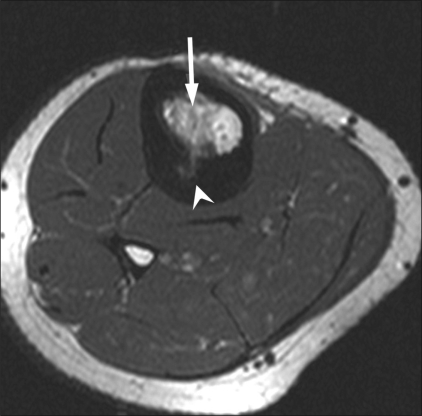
Osteoid osteoma of the tibia. Postprocedure T1W axial MRI shows the zone of ablation (arrow) surrounding the ablated nidus (arrowhead)
